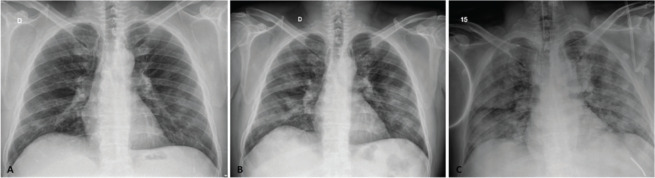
Chest radiographs, posteroanterior views (A and B) and in bed (C), performed at intervals of 2 days, show progression of the diffuse and bilateral pulmonary opacities. Note the tracheal cannula and other vital devices (C).
